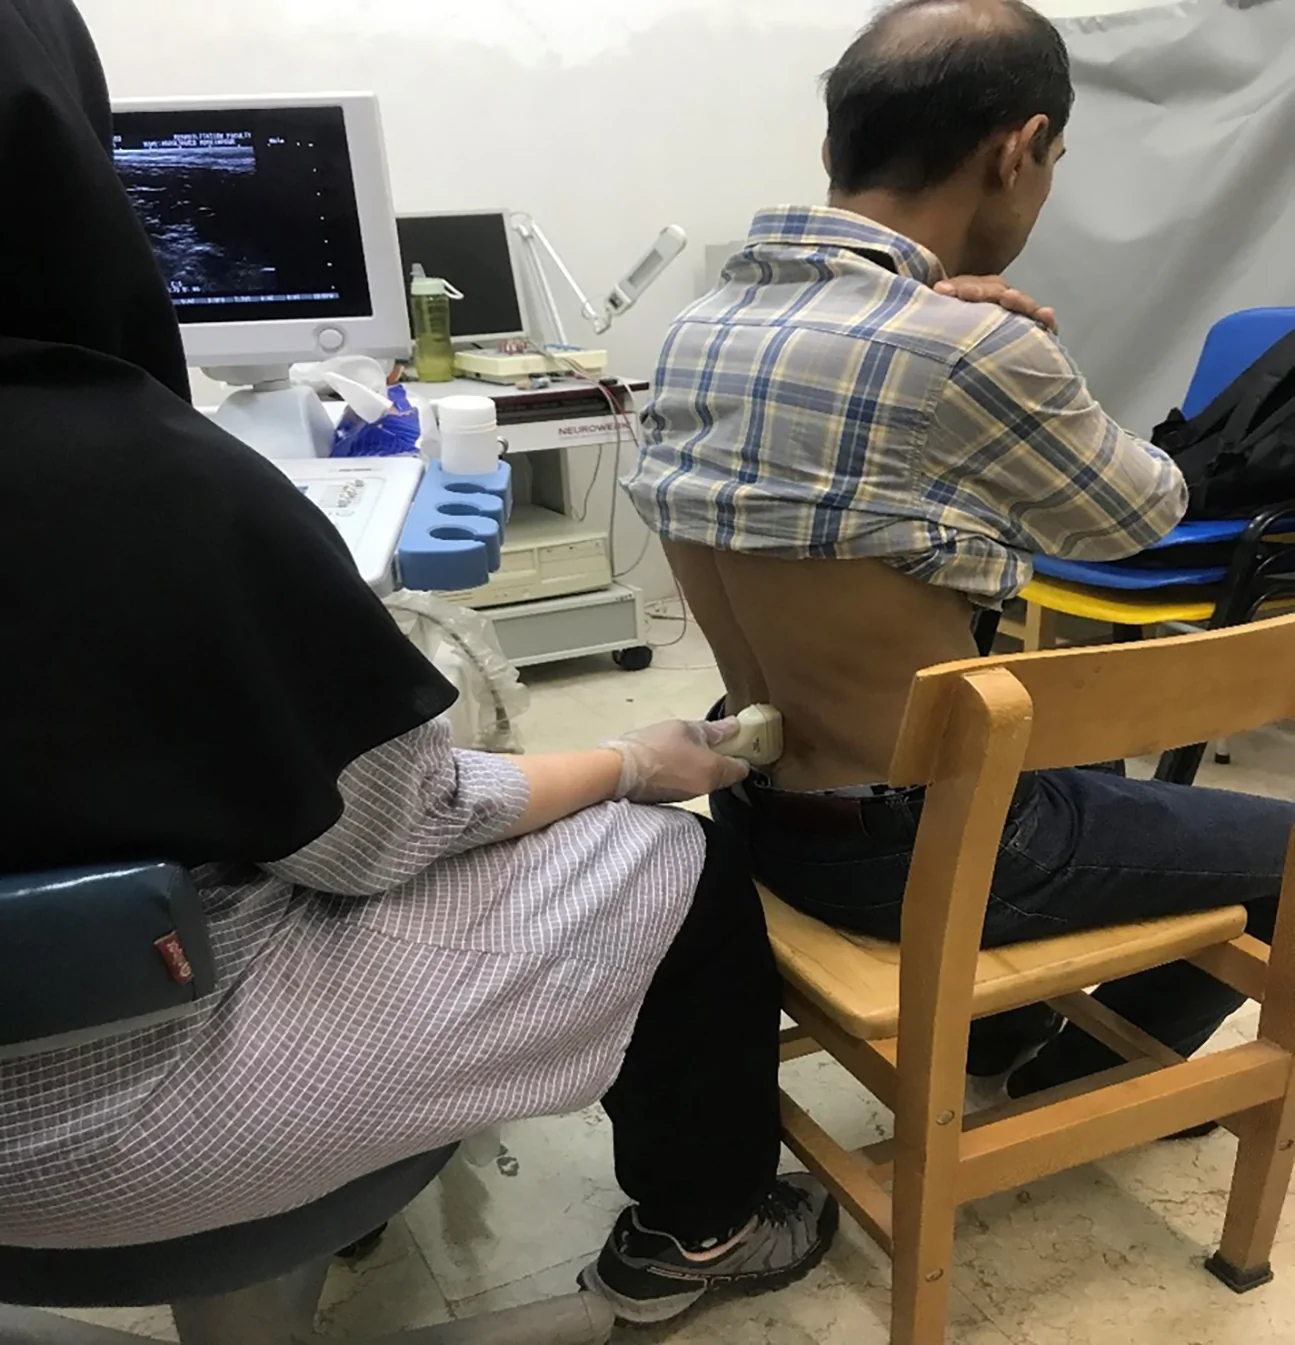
Ultrasound image showing Multifidus muscle thickness in the sitting position. Created by the authors. Published under CC BY 4.0.
